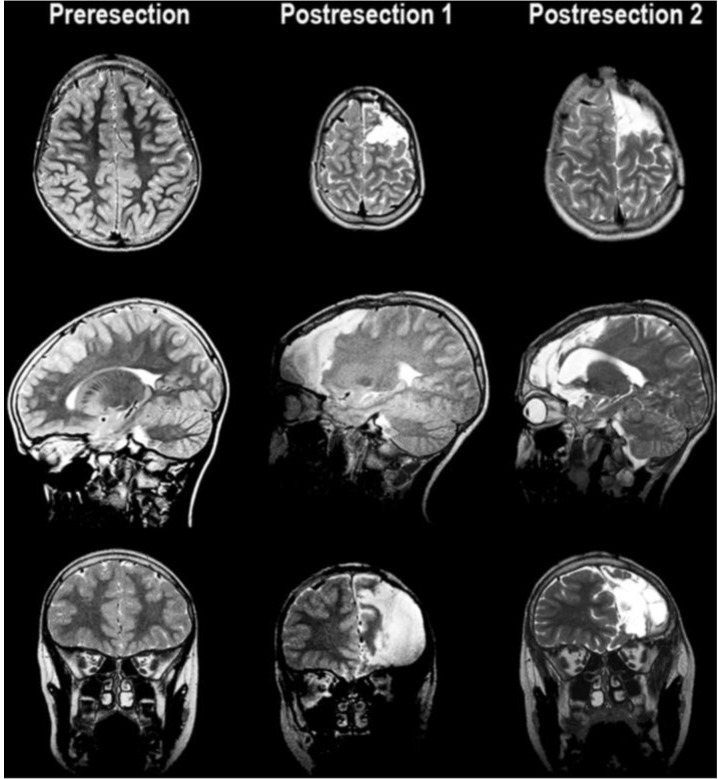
MRI images showing pre-resection (*left column*), and resection cavity following the first procedure (*middle column*) with resection to the pre-motor gyral bank and second procedure (*right column*) with resection back to the primary motor area.
